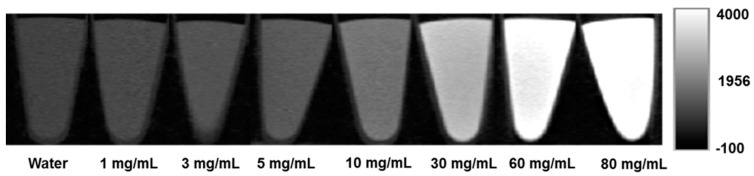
Coronal slices of CT images of AuD suspension at different concentrations.
